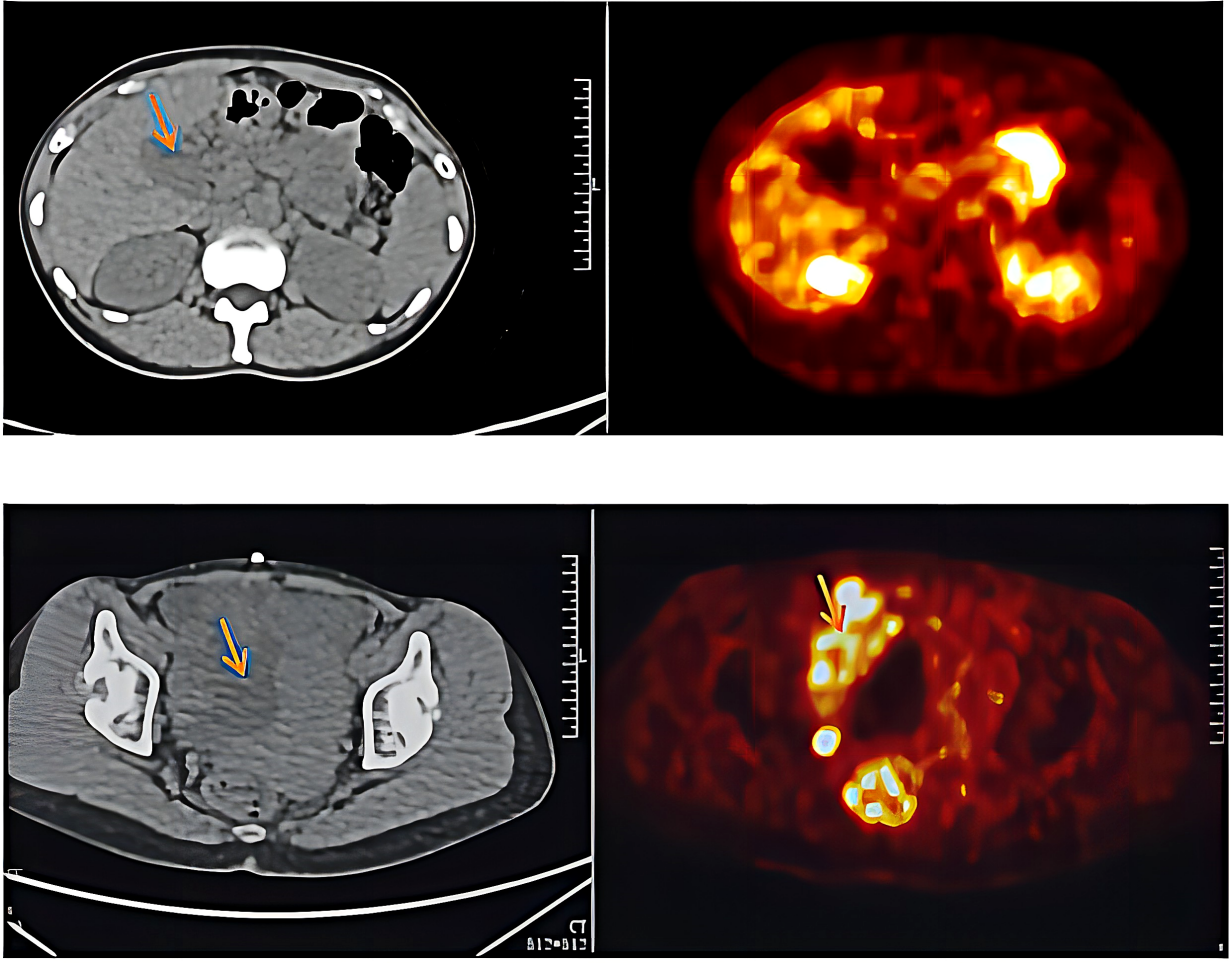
Results for PET-CT examination after chemotherapy and targeted therapy (March 10^th^, 2021).
